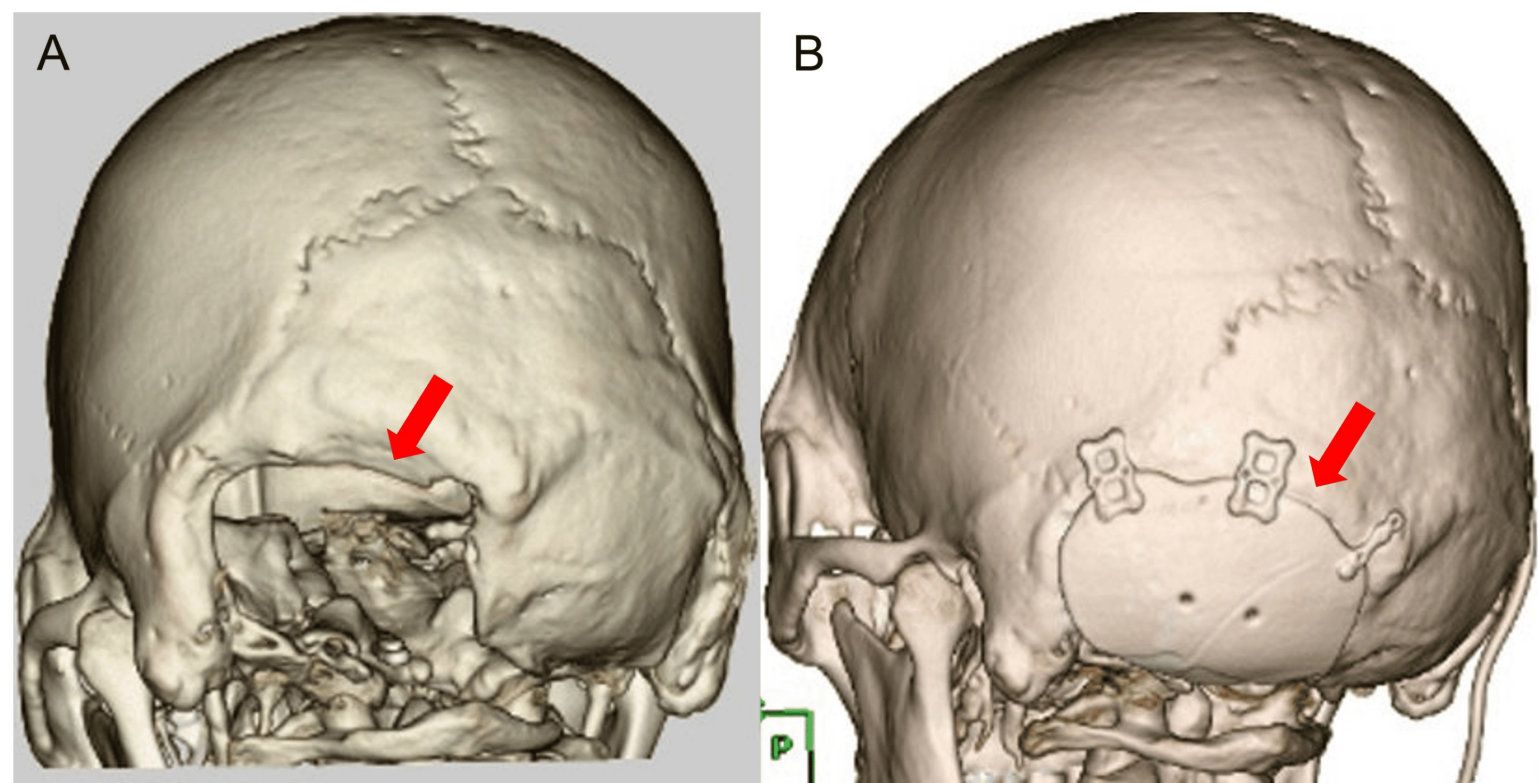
Preoperative 3D bone imaging and postoperative reconstruction. (A) Preoperative 3D CT of the skull demonstrating the left posterior fossa defect (arrow). (B) Postoperative CT after posterior fossa cranioplasty with a synthetic implant secured using titanium plates (arrow).
